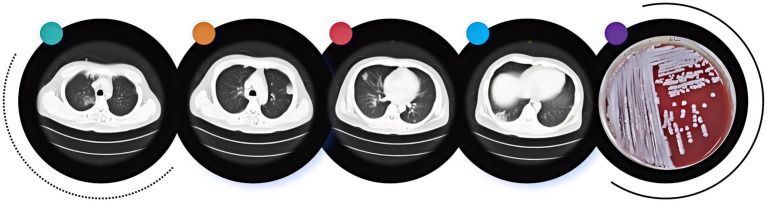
CT imaging and sputum culture of a typical case of pneumonia due to KP.
